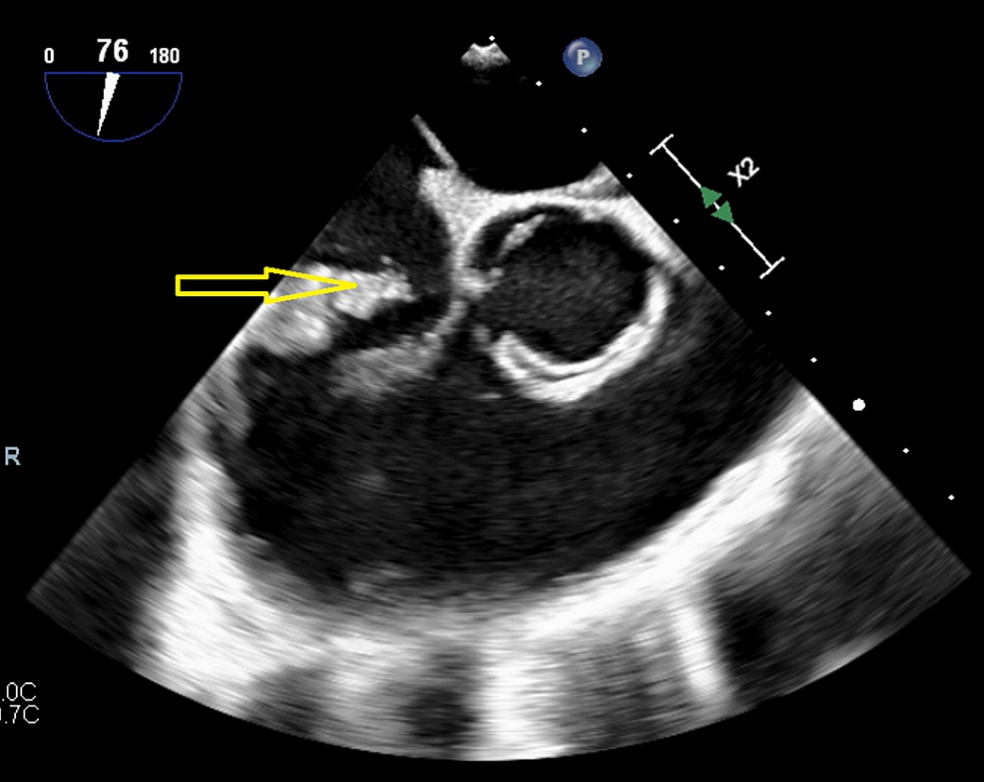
Short-axis view of TEE showing large vegetation attached to the anterior leaflet of the tricuspid valve (arrow) TEE: transesophageal echocardiogram
